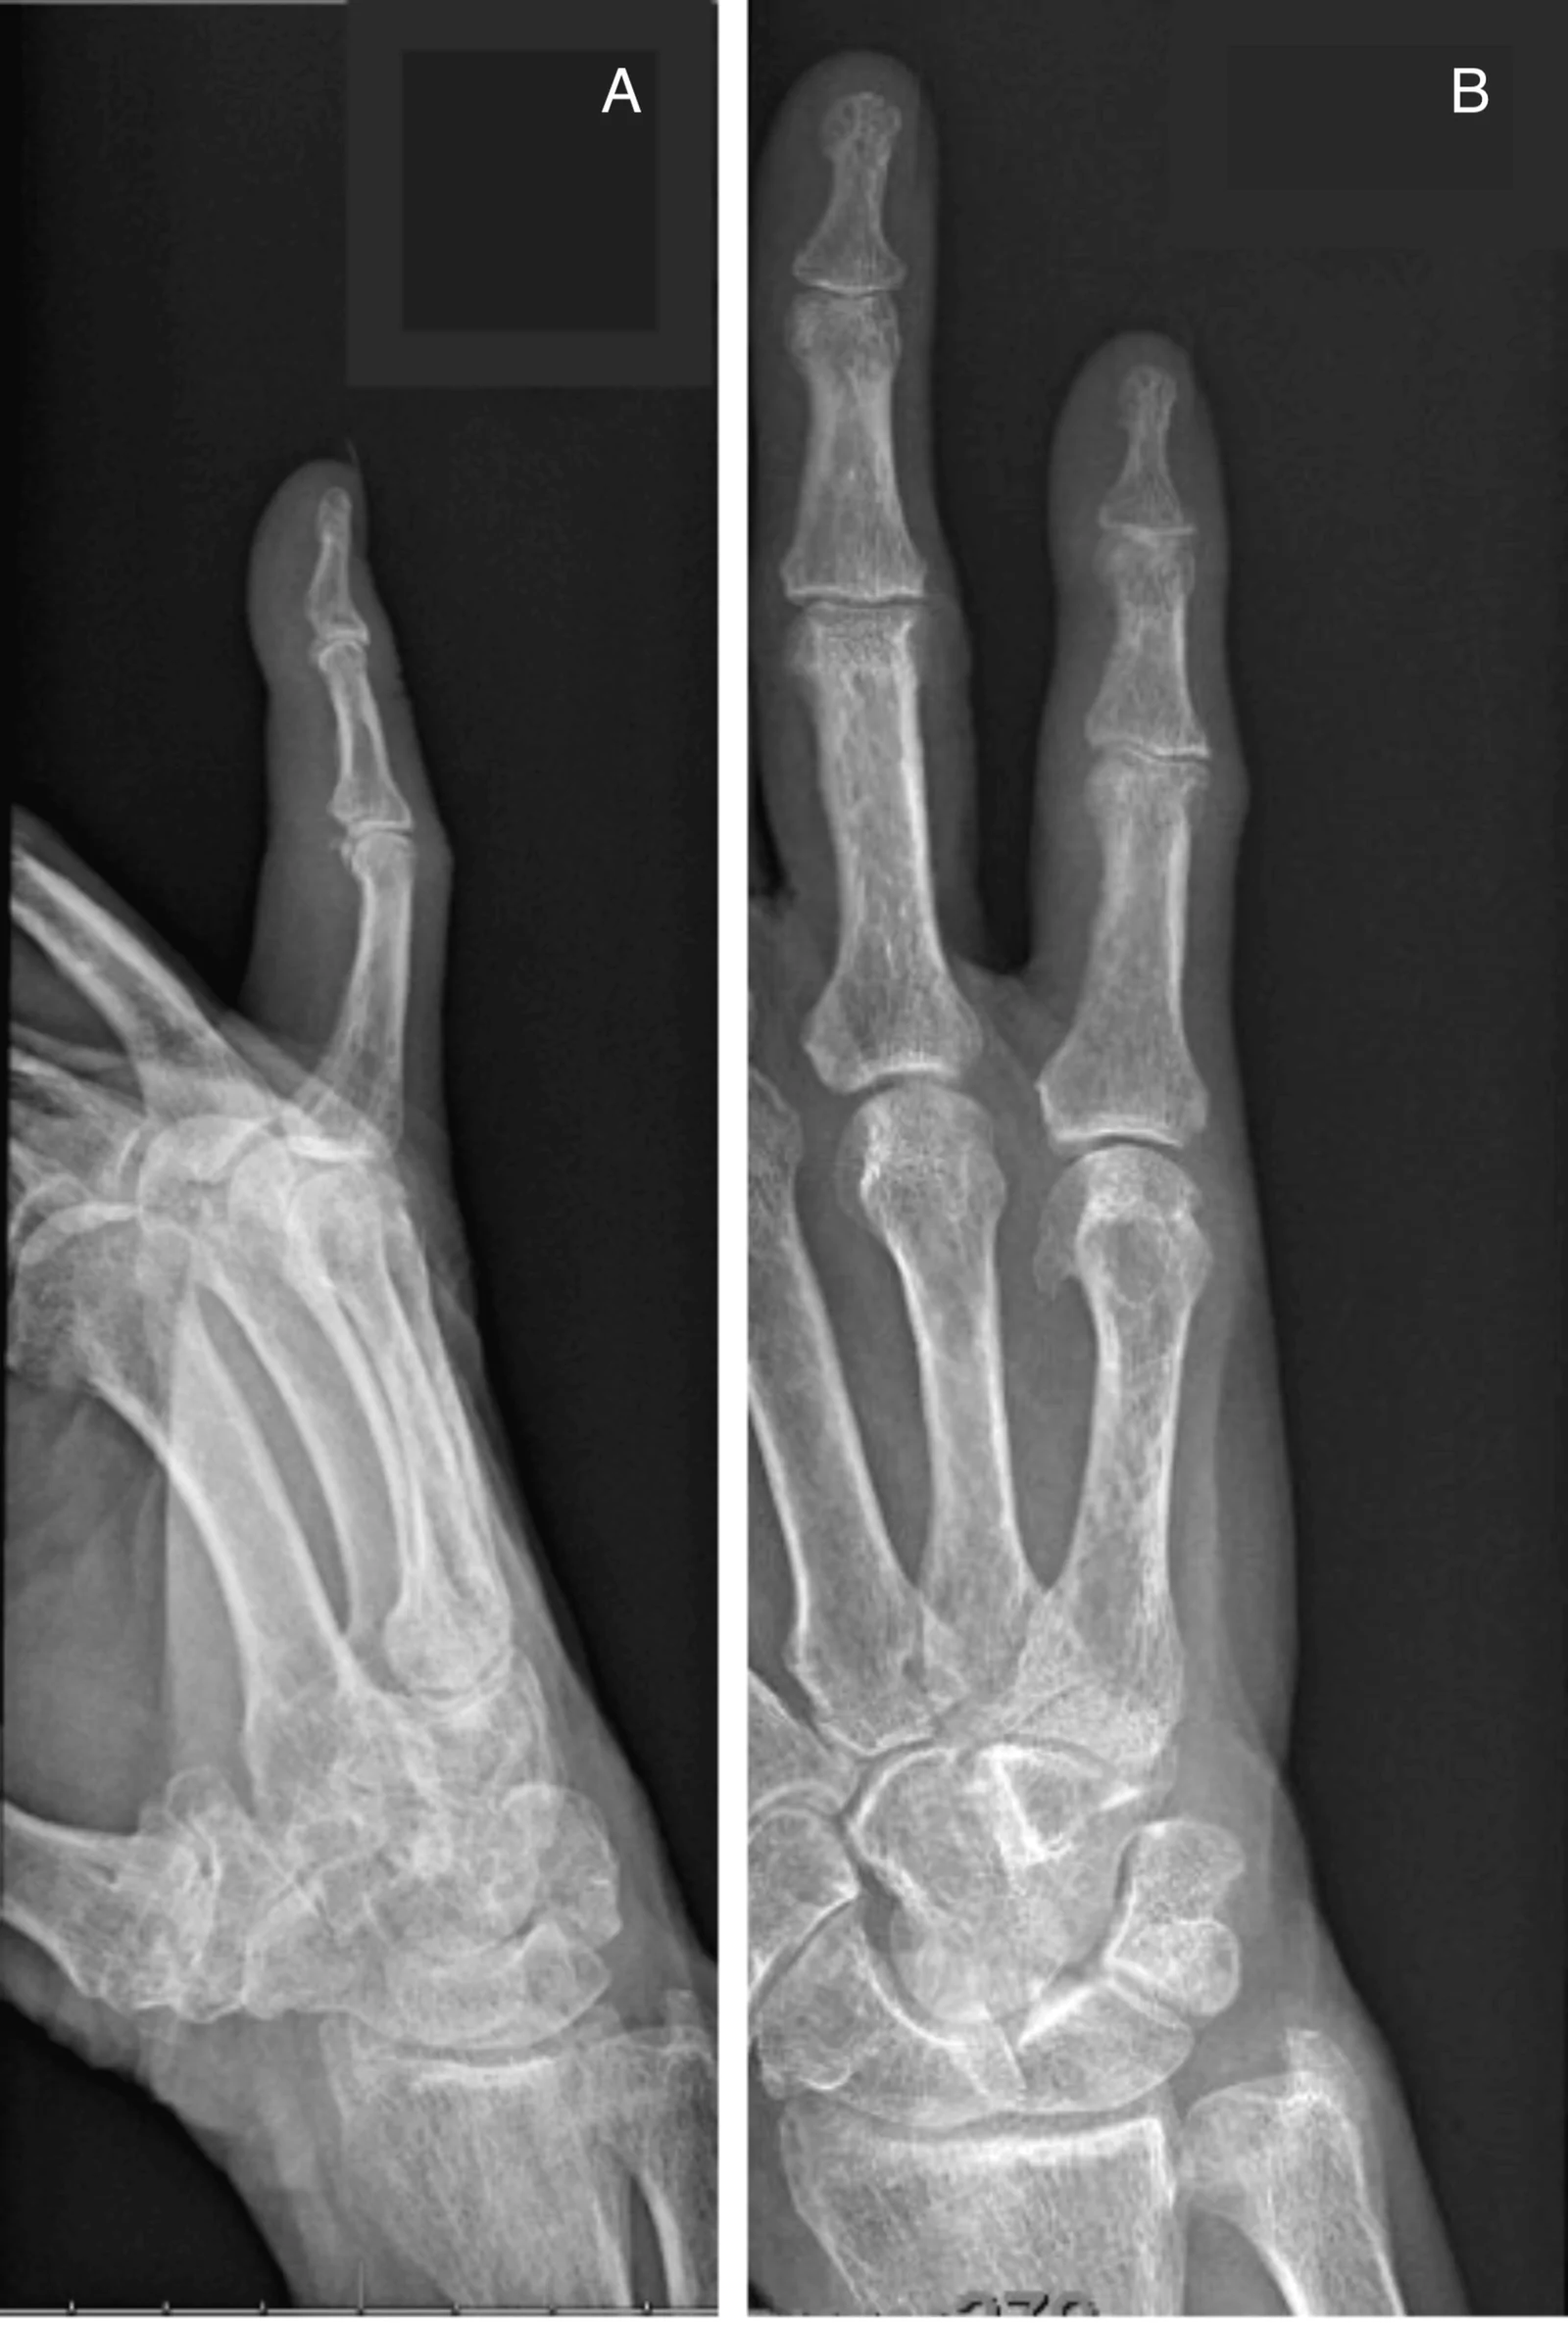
Lateral (A) and anteroposterior (B) X-ray at the two-month follow-up appointment.
